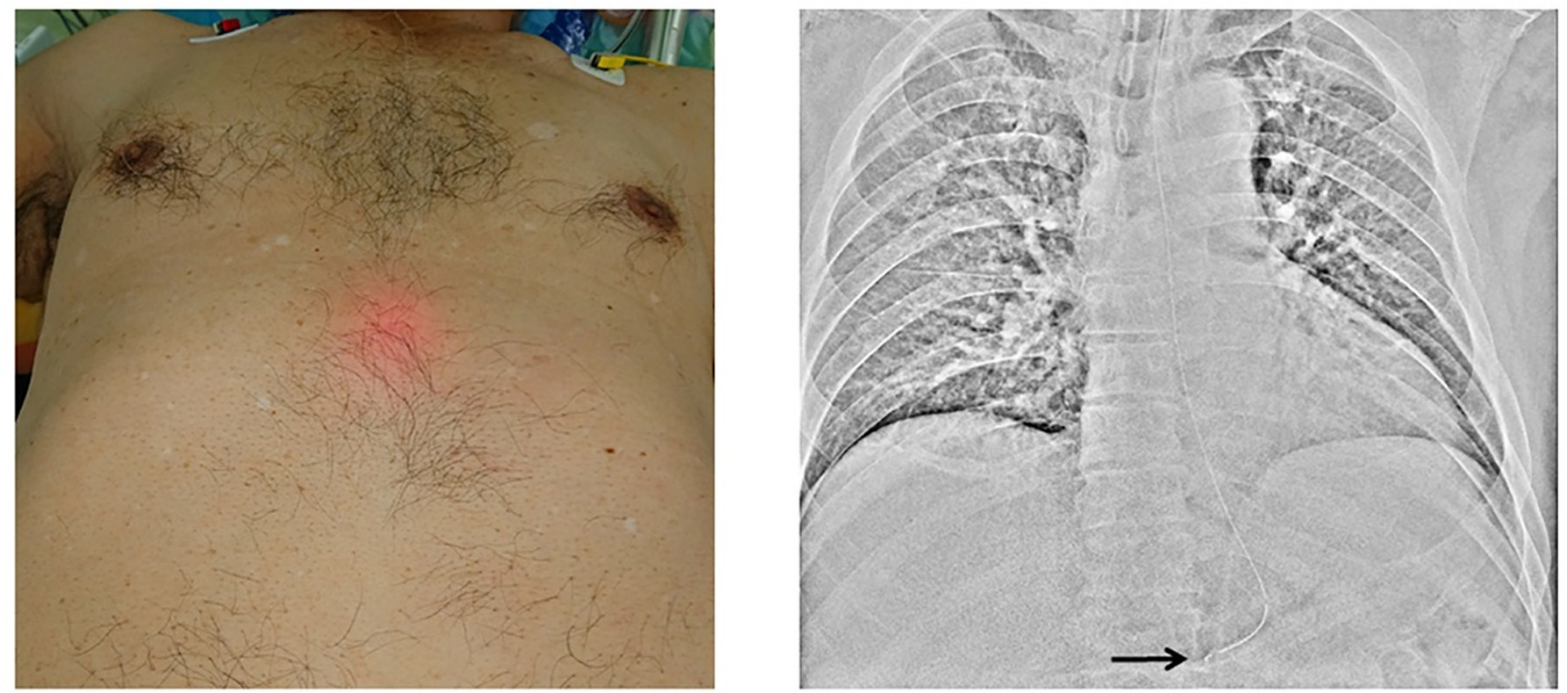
Positive biologically transparent light sign in the central epigastric area and corresponding X-ray image. The arrow indicates the tip of the nasogastric tube.
